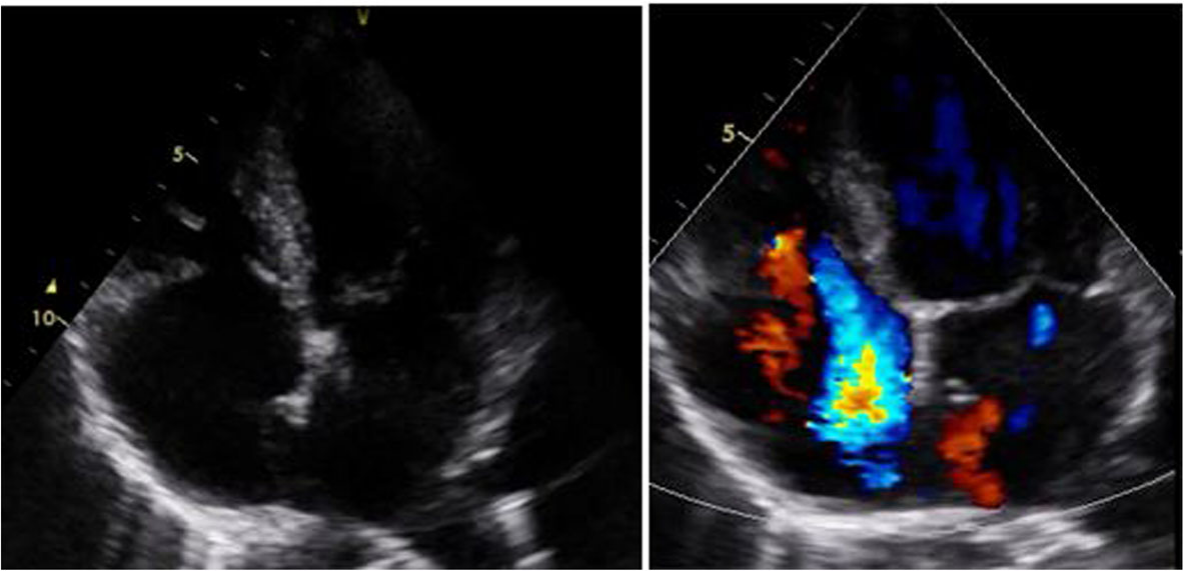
Transthoracic echocardiogram apical four-chamber view showed thickened and retracted tricuspid valves with significant regurgitation and patent foramen ovale
